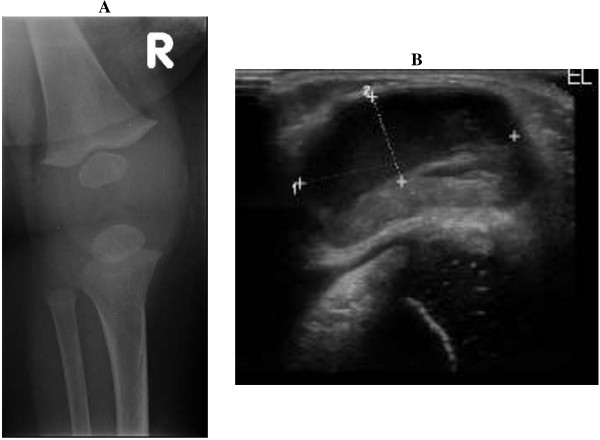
Radiological images of the lesion 3 weeks after administering the 13-valent pneumococcal conjugate vaccine.*PanelA:* Right knee X-ray showing anterior fluid collection. *PanelB:* Ultrasound of the right knee showing swelling (measuring 2.9cm transversely and 1.5cm anteroposteriorly) of the anterior aspect of the knee due to fluid collection.
